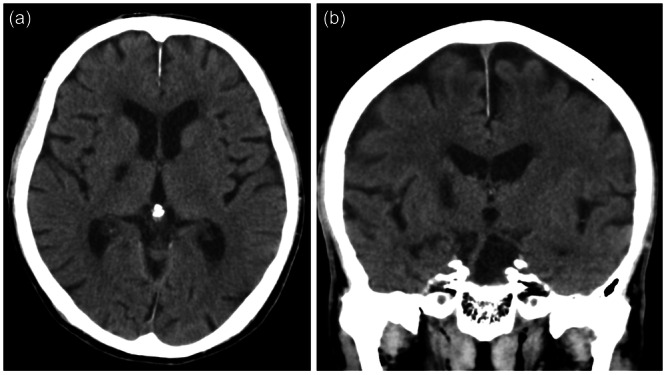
(a) Axial and (b) coronal computed tomography images taken 1 month after infarction.
